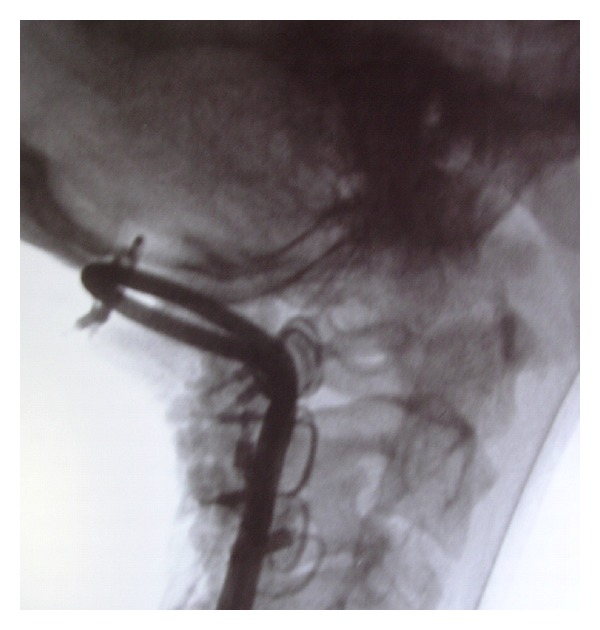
Immediate postoperative radiographs showing the occipitocervical fusion.
